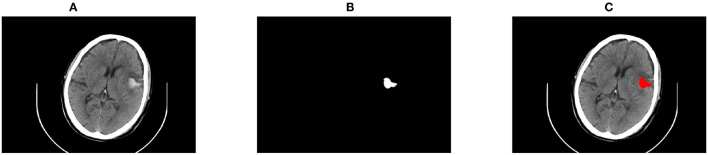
Results of the second region growing segmentation. (A) The original CT image; (B) Segmentation of the second region growing; (C) Restoration of the second region growing results.
